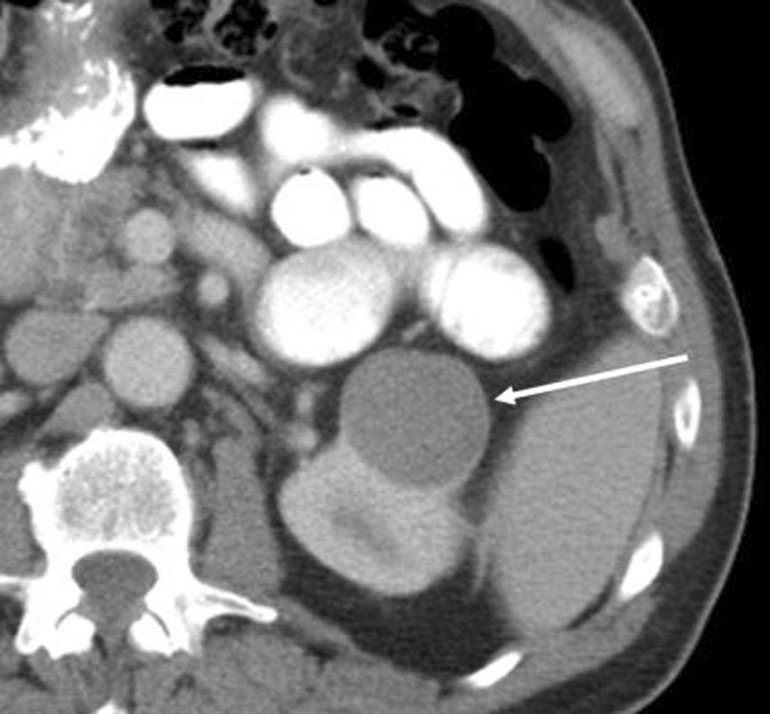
A 64-year-old man with papillary RCC of the left kidney. The nephrographic phase of the axial CE-CT demonstrates a homogeneously low attenuation mass (*arrow*)
